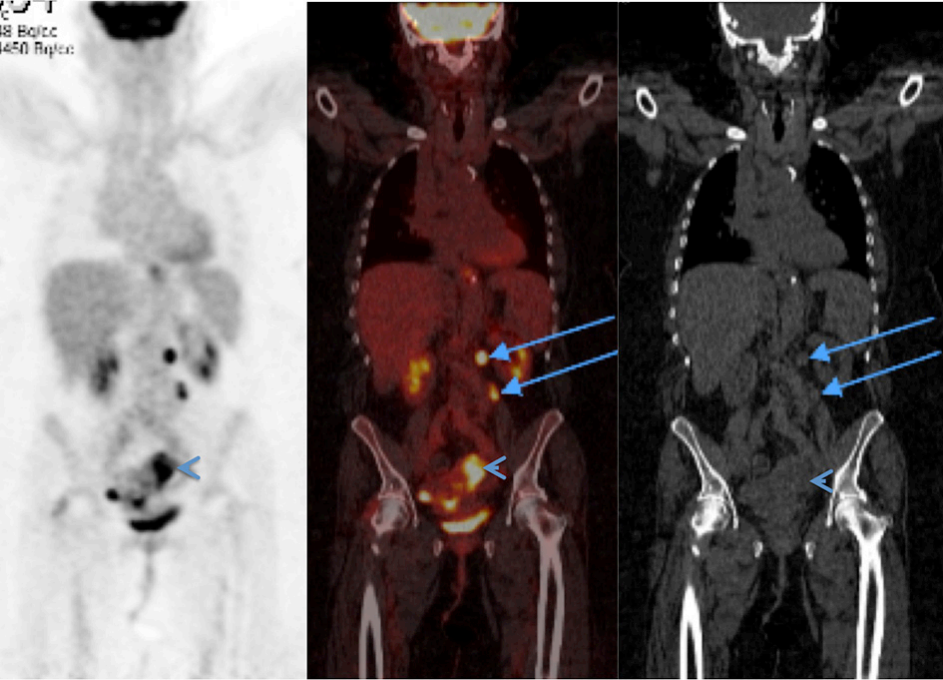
A 68-year-old female with Grade 3 endometriod endometrial carcinoma. Staging CT demonstrated suspicious left para-aortic nodes (arrows). A PET CT was performed demonstrating avid FDG tracer uptake in the nodes confirming metastases (arrows), in keeping with FIGO Stage IIIC2 endometrial carcinoma. The open arrow highlights the endometrial carcinoma, which also has avid FDG uptake. FDG, fluoro-2-deoxy-D-glucose; PET CT, positron emission tomography.
